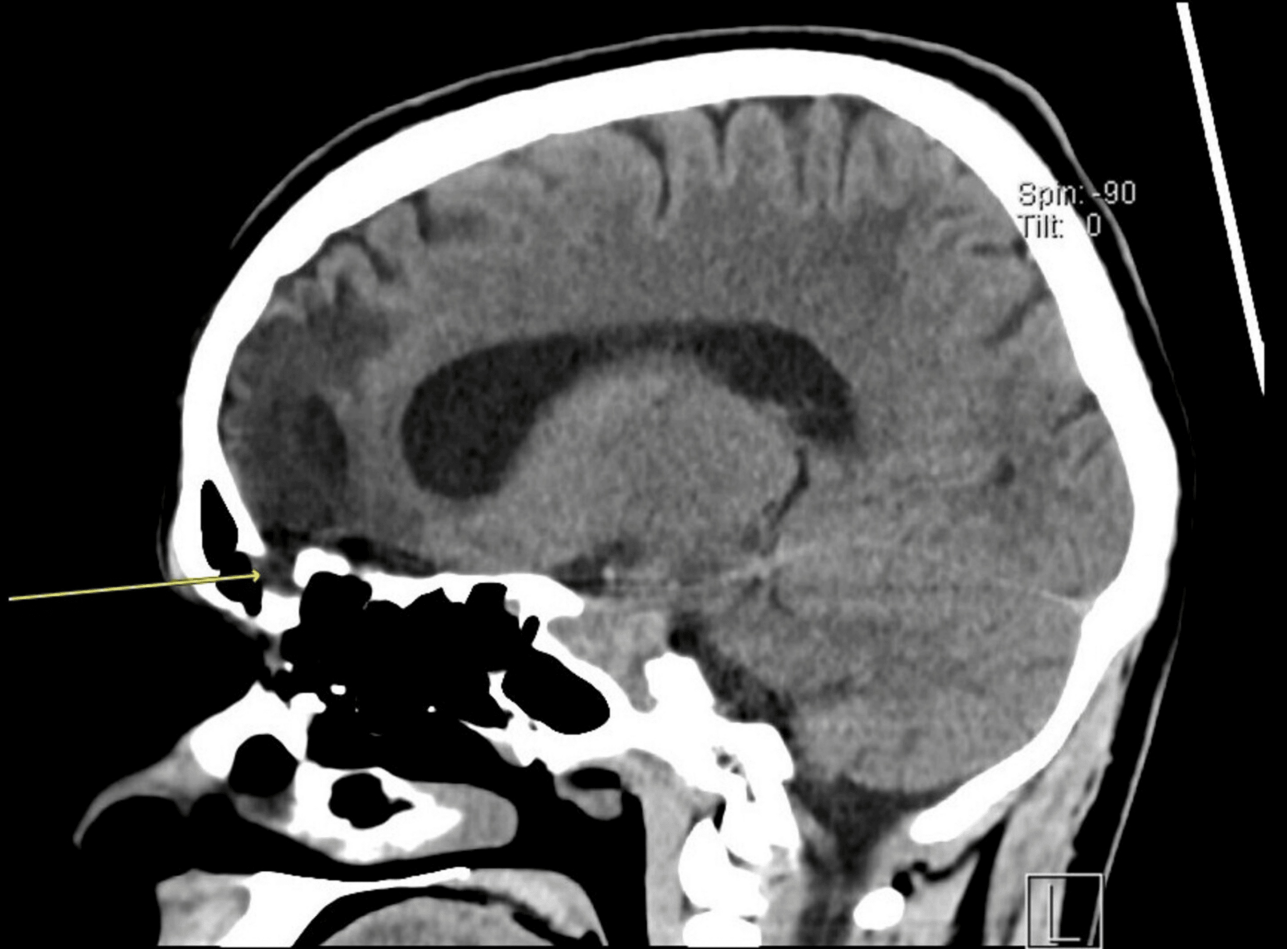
CT scan’s sagittal view showing a communicating defect and an encephalocele (arrow).
